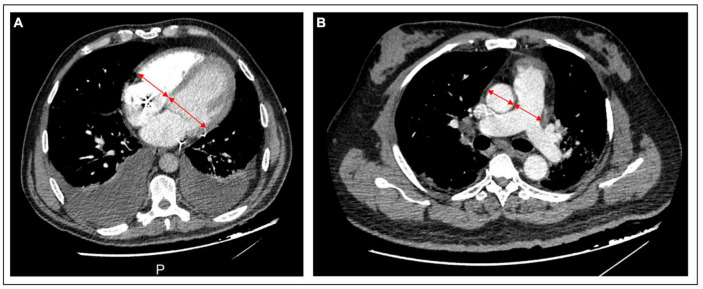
CTPA images of cardiac chambers and vessels measurement. (A) RV and LV basal diameters are measured by the maximum distance in diastole between the ventricular endocardium and the interventricular septum in a 4-chamber axial view. (B) In the axial view, the pulmonary artery and aorta diameter are measured by the broadest transverse diameter (inner wall-to-inner wall). CTPA, computed tomography pulmonary angiogram; LV, left ventricle; RV, right ventricle.
